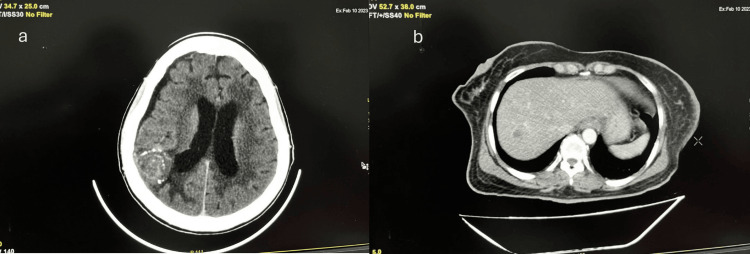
(a) A 35 x 28 mm lesion in the right post-parietal lobe with perilesional edema suggestive of stable disease via CECT; (b) A 12 x 12 mm small non-enhancing hypodense lesion in the right lobe in segment VII suggestive of a stable lesion observed on CECT. CECT, contrast-enhanced computed tomography
